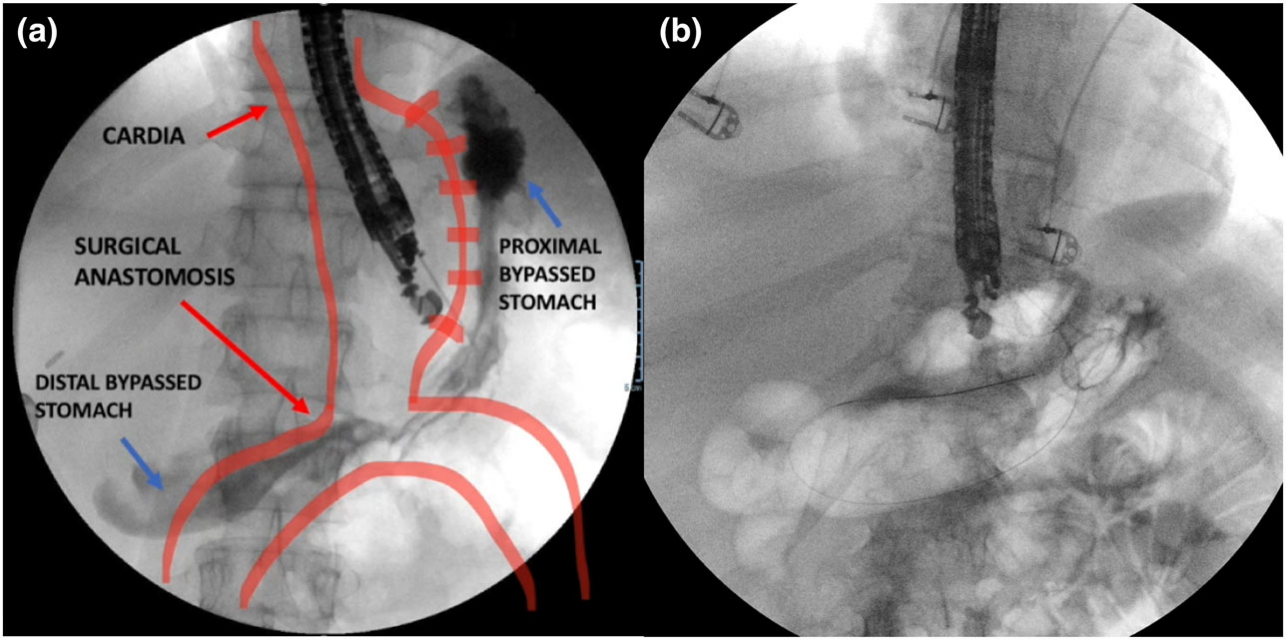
(a) First endoscopic ultrasound (EUS) puncture of the middle stomach with opacification to understand the postsurgical anatomy in a patient with a gastric bypass to decide the better location of the subsequent EUS‐guided gastro‐gastric anastomosis. (b) Of note, the proximal and distal bypassed stomach are more dilated compared to the middle gastric region, but this location was preferred to have a gastro‐gastric oblique axis, ensuring an easy passage for further transgastric interventions.
